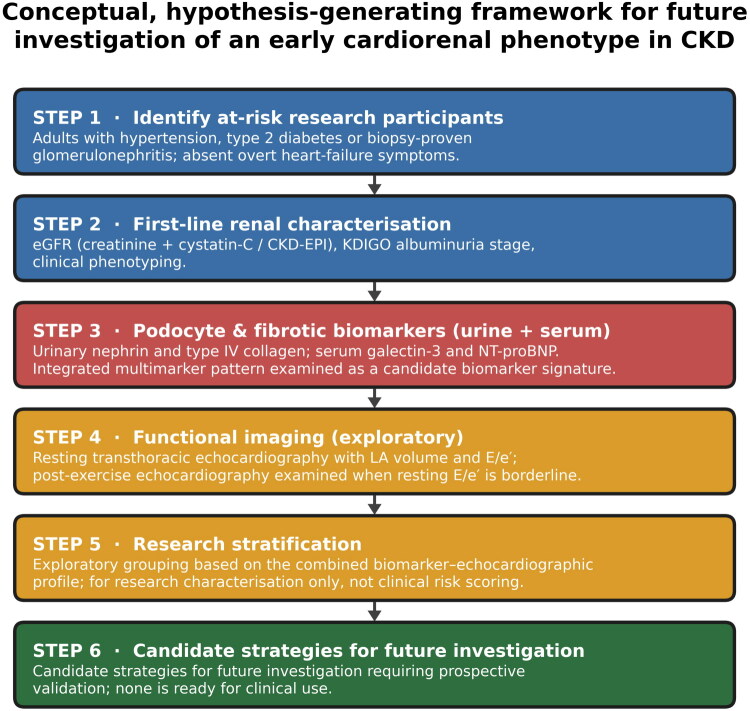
Conceptual framework for future investigation of an early cardiorenal phenotype in CKD (exploratory and hypothesis-generating; not a validated screening algorithm and not recommended for clinical implementation without prospective external validation). A six-step conceptual framework comprising identification of at-risk research participants (step 1), first-line renal characterization (step 2), the podocyte–fibrotic biomarker panel examined as a candidate biomarker signature (step 3), resting and post-exercise echocardiography (step 4), exploratory research stratification based on the combined biomarker–echocardiographic profile (step 5) and candidate strategies for future investigation requiring prospective validation (step 6). The framework is offered as hypothesis-generating only; it is not a clinical decision-support tool or a recommended management pathway and each component needs to be prospectively validated before use in the clinic.
